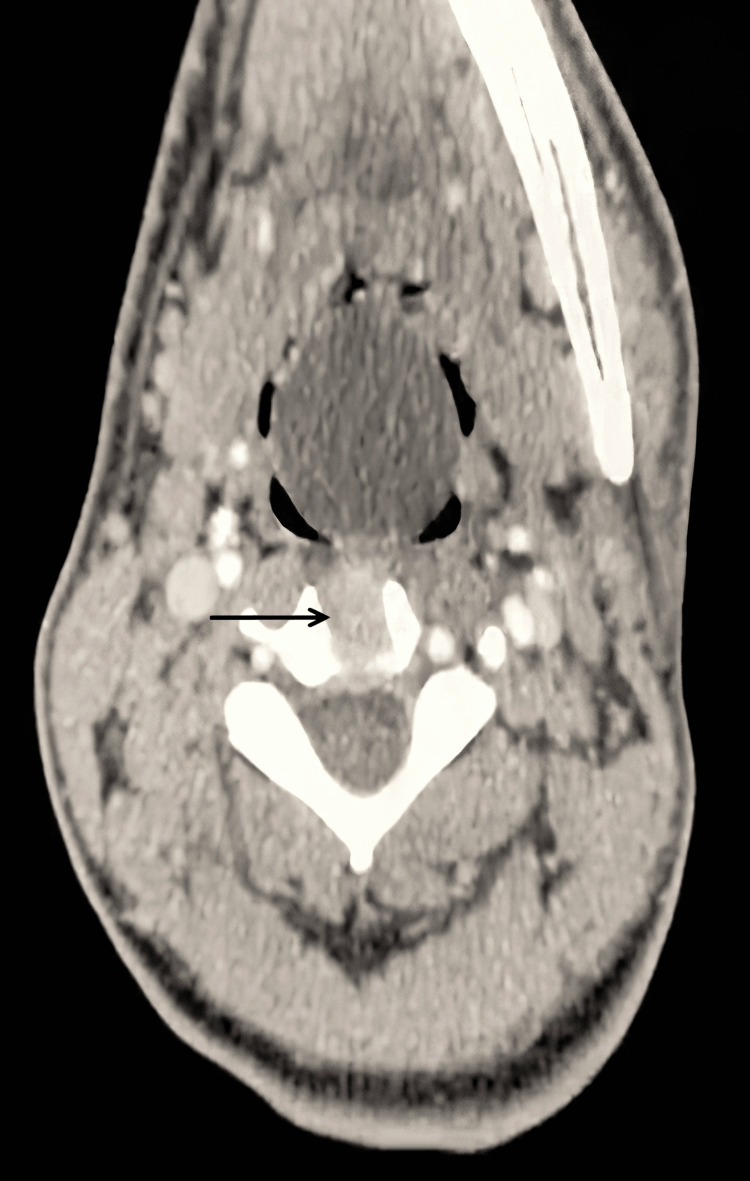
Axial neck CT scan at the hypopharynx level.
